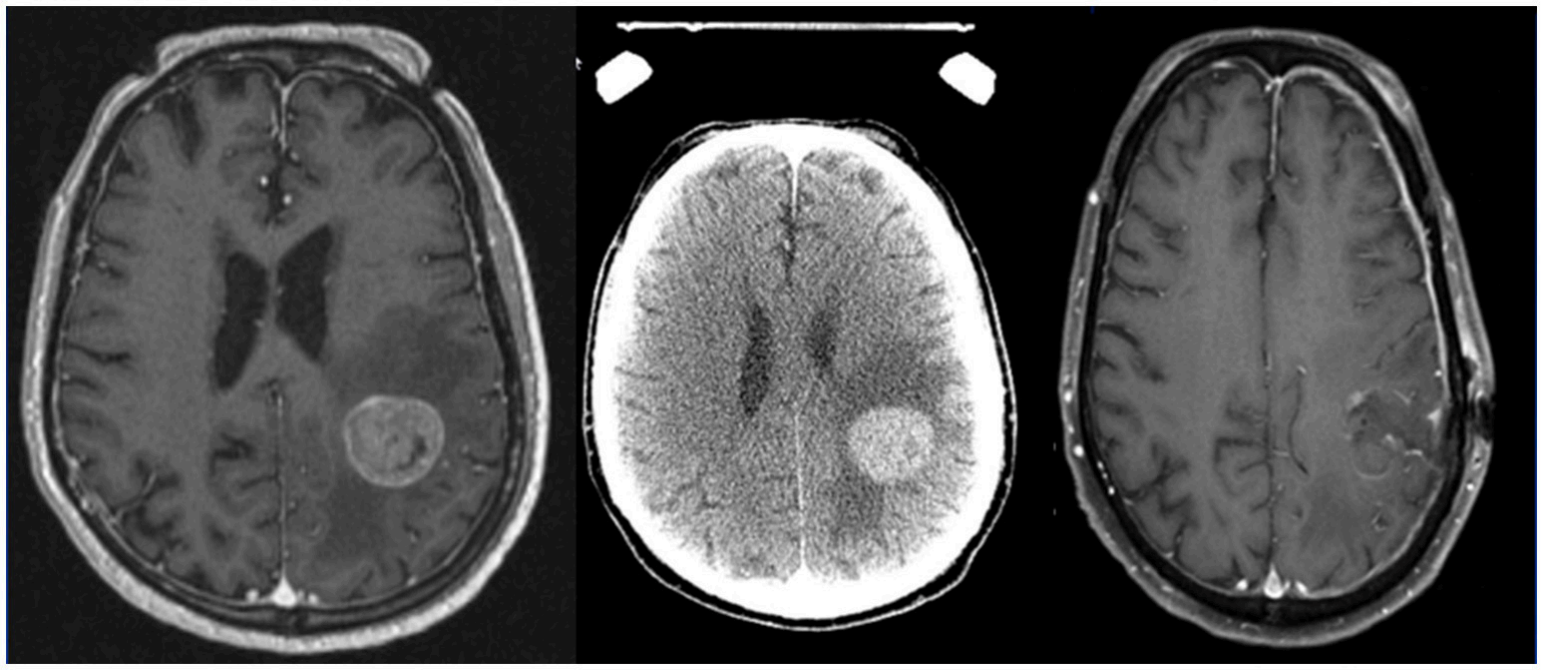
Axial MRI and CT images of a patient with a brain metastases treated with preoperative stereotactic radiosurgery followed by resection days later.
